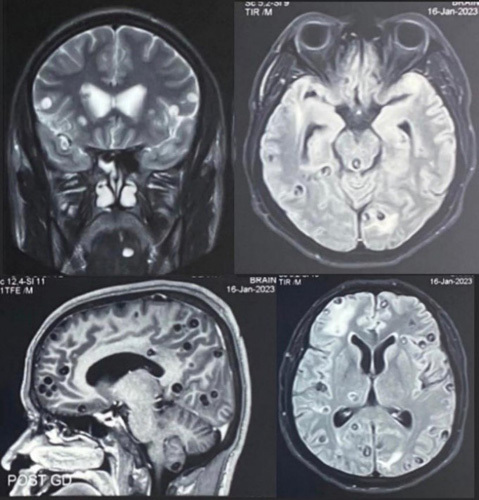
MRI brain showing innumerable cystic lesions throughout cerebral hemisphere and within tongue muscles giving starry sky appearance.
